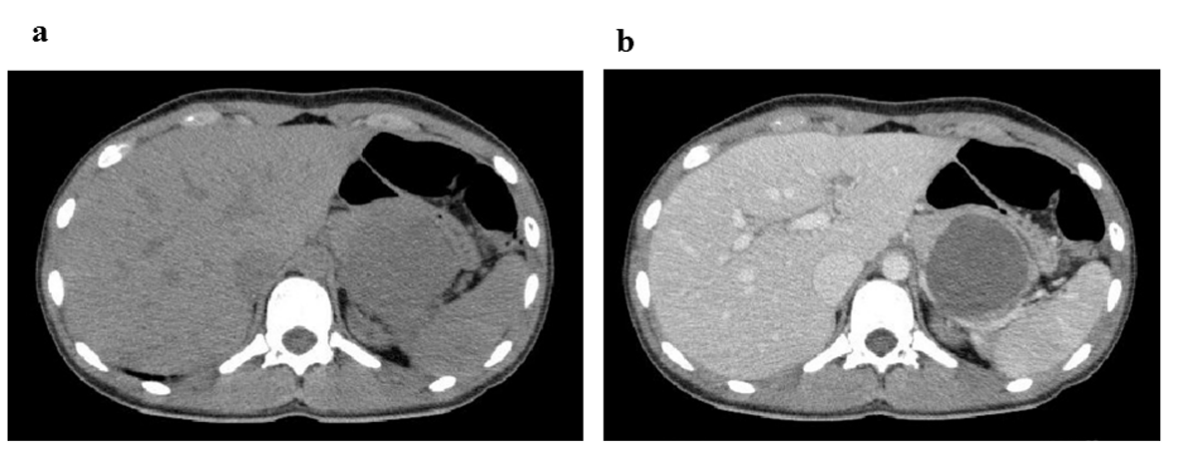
CT images at 4 h after abdominal injury. (a) Plain CT reveals a cystic mass measuring 55 mm in the pancreatic body and tail. (b) Contrast-enhanced CT indicates a heterogeneous internal density and smooth rim enhancement of the mass.
